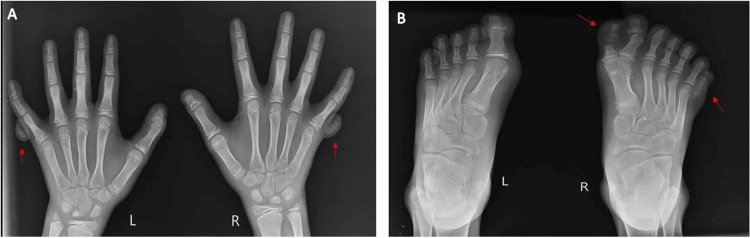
Case 2: (A) and (B) showing X-rays of the right and left hands and feet, confirming polydactyly
